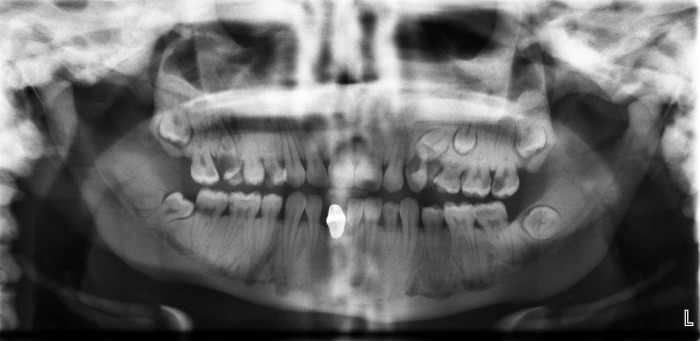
Right hand posteroanterior (PA) radiograph showed hyperdense bones, particularly in the carpals, radius and ulna. Obliteration of cancellous bones, resulting in the loss of corticomedulary demarcation was observed. A previous fracture of the proximal phalanx of the first finger was also noted. The other radiographic finding of the hand x-ray was the presence of “bone-within-bone” appearance in metacarpal and phalangeal bones (Figure 5, A). In addition, the former anteroposterior (AP) and lateral views of the tibia and fibula demonstrated generalized osteosclerosis and multiple previous fractures (Figure 5, B).
